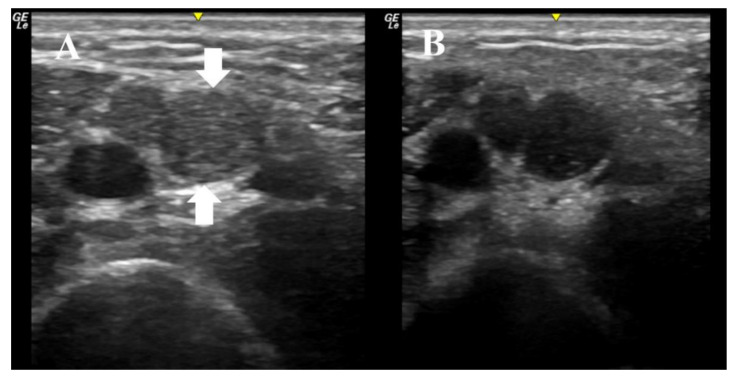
These ultrasound images were obtained with a 22 MHz linear array transducer, and they depict the median nerve in the right arm of an 11-year-old boy with subacute distal weakness and sensory loss; (A) demonstrates imaging with the transducer at 90 degrees to the nerve. The nerve has two large fascicles, one between the arrows and the other directly to the left of the largest fascicle. The area of the nerve at this site is 20 mm^2^. (B) demonstrates the same image, but the transducer is at an angle just slightly off 90 degrees, which demonstrates the anisotropy of the nerve at this site, as the fascicles become hypoechoic with just a minor alteration of the angle of insonation. This boy was treated with IVIG and his strength normalized.
